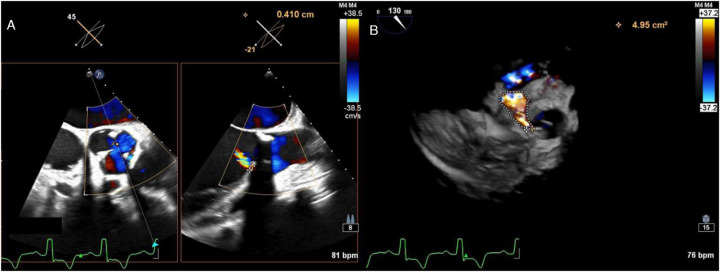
Transesophageal echocardiography identified the leakage orifice at the original right coronary cusp position, measuring 4.1 mm in width (A), with a regurgitant jet area of 4.95 mm² (B).
